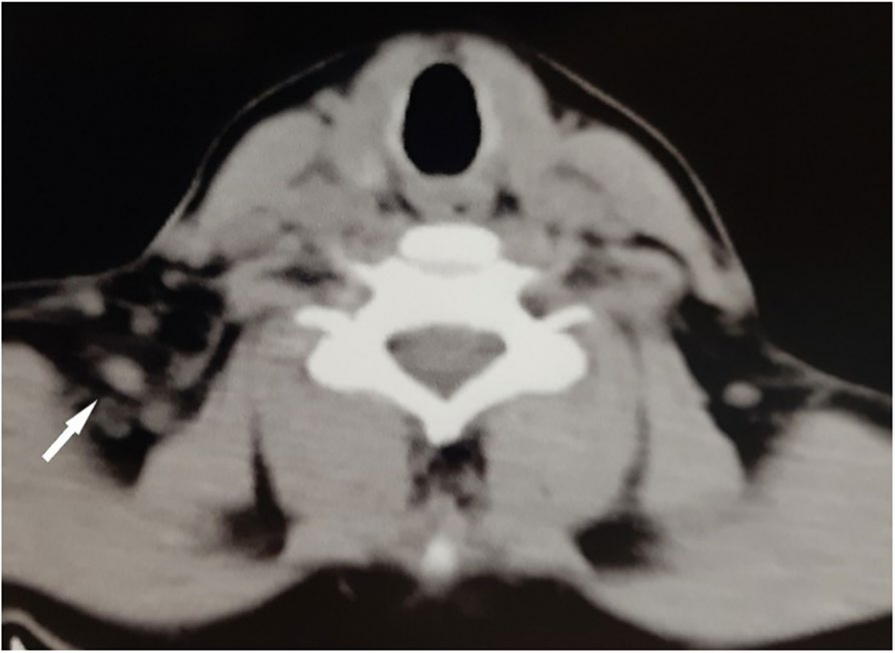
CT scan of the neck after delivery. Multiple lymph nodes (LN) on the right posterior triangle with edematous adipose contour
